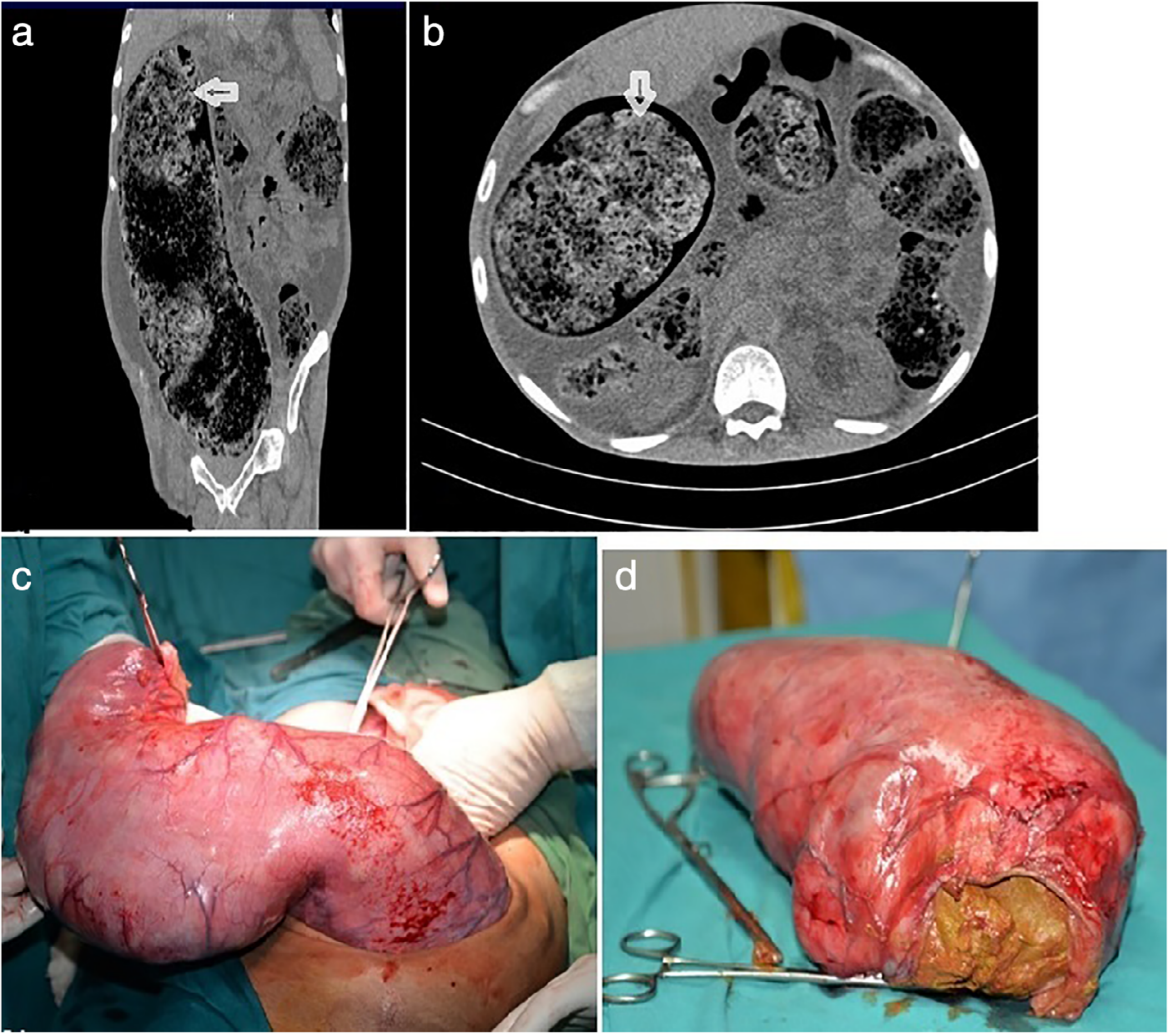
(a) Computed tomography (CT) abdomen showing dilated sigmoid colon, filled with a hyperdense mass with air foci, shown by arrow; (b) CT abdomen (transverse section) demonstrated dilated large bowel, filled with hyperdense material; (c) intraoperative findings showed markedly dilated left‐sided colon, with approximate diameter of 30 cm; and (d) resected sigmoid colon filled with fecal matter and fecaliths.
